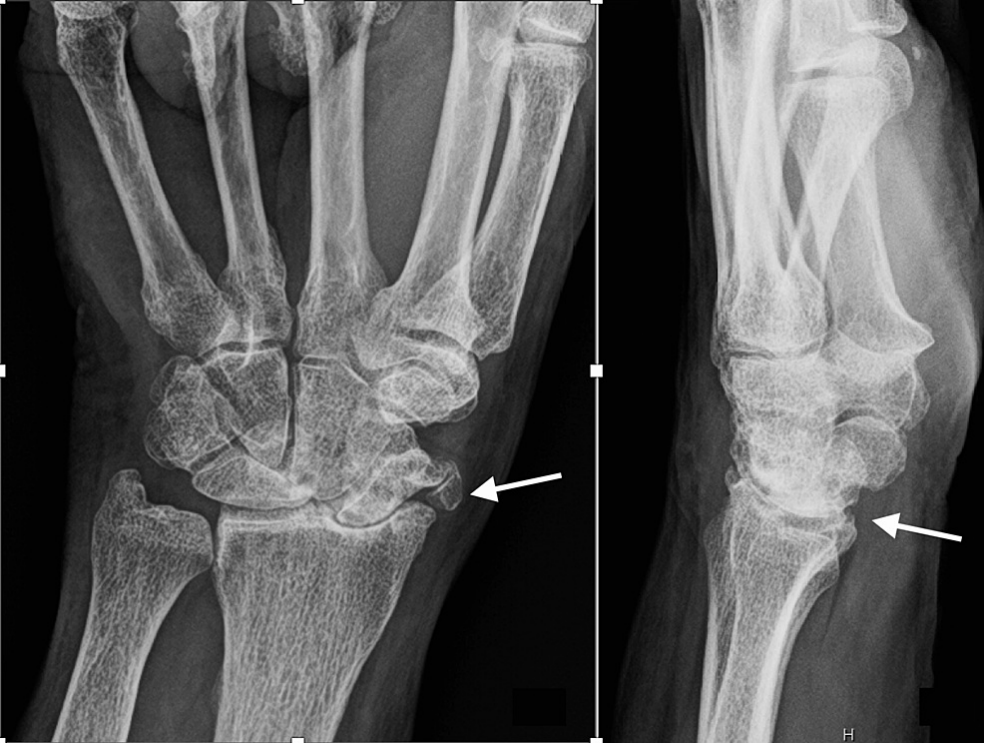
Preoperative radiographs of Case 1 Posteroanterior and lateral views of a 78-year-old woman with chronic post-traumatic scapholunate advanced collapse (SLAC). The white arrows show radiocarpal arthritis.
